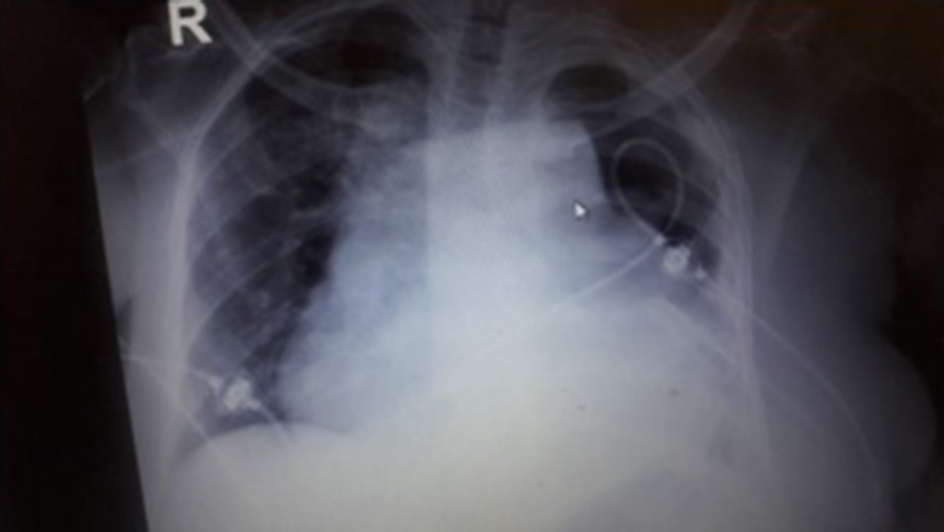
Chest x-ray of the patient showing left-sided hydro-pneumothorax.
